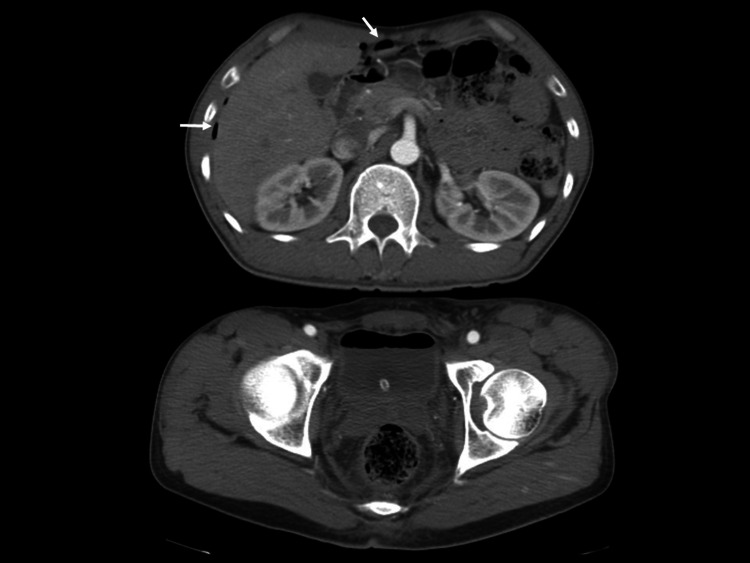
Follow-up computed tomography (CT) findings on day 3 Follow-up CT on day 3 revealed multiple free air pockets in the intra-abdominal cavity (arrow) and the disappearance of the fluid collection and trabecular formation in the bladder.
